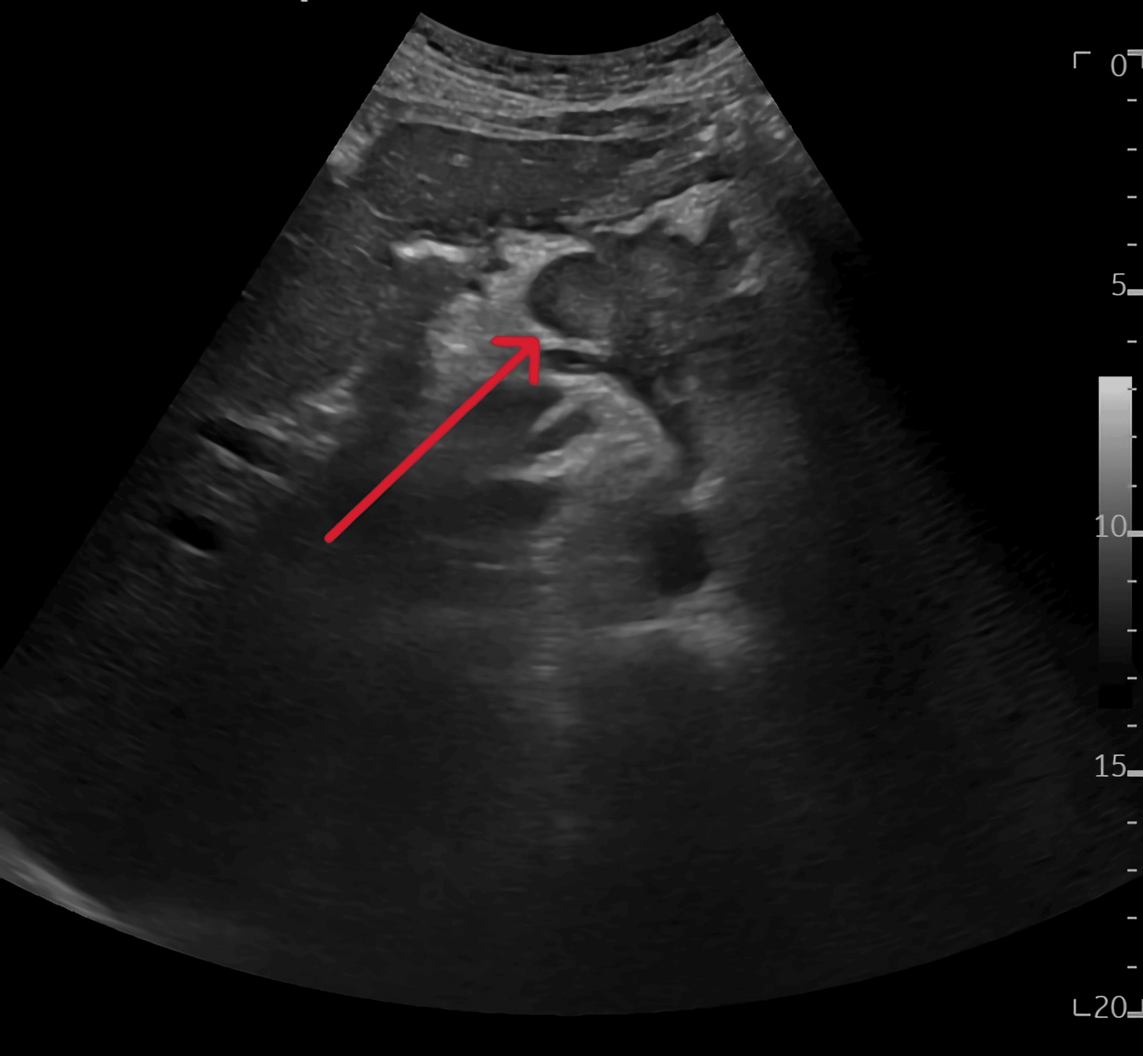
Abdominal ultrasound Solid mass with irregular contours and heterogeneous characteristics
